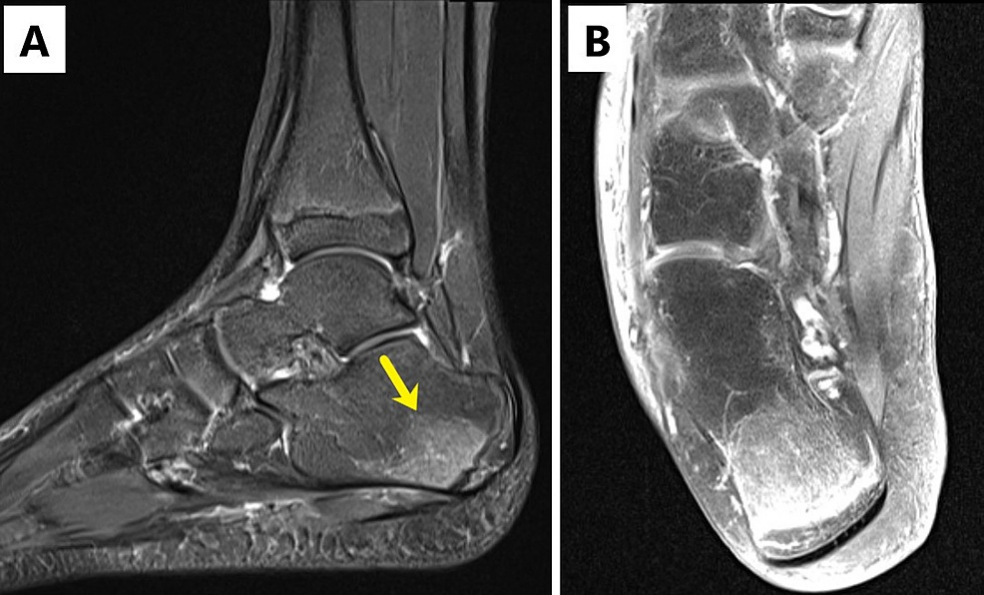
Magnetic resonance imaging (MRI) of the right ankle and foot. (A) Sagittal inversion recovery demonstrating increased signal intensity within the calcaneus (yellow arrow). (B) Coronal T1-weighted fat saturation, postgadolinium contrast demonstrating enhancement in the area of concern, suggestive of osteomyelitis.
